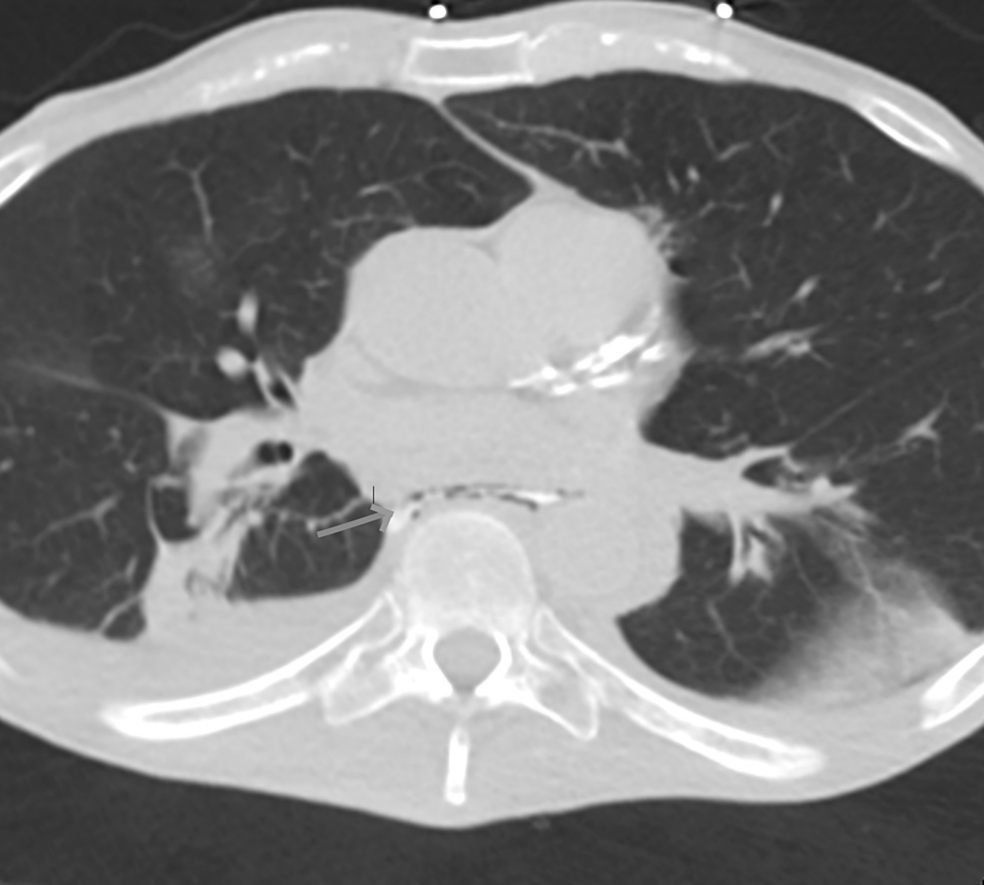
This axial computed tomography image demonstrates pneumomediastinum and free flow of the enteric contrast material into the mediastinum outside the esophageal lumen compatible with esophageal tear
